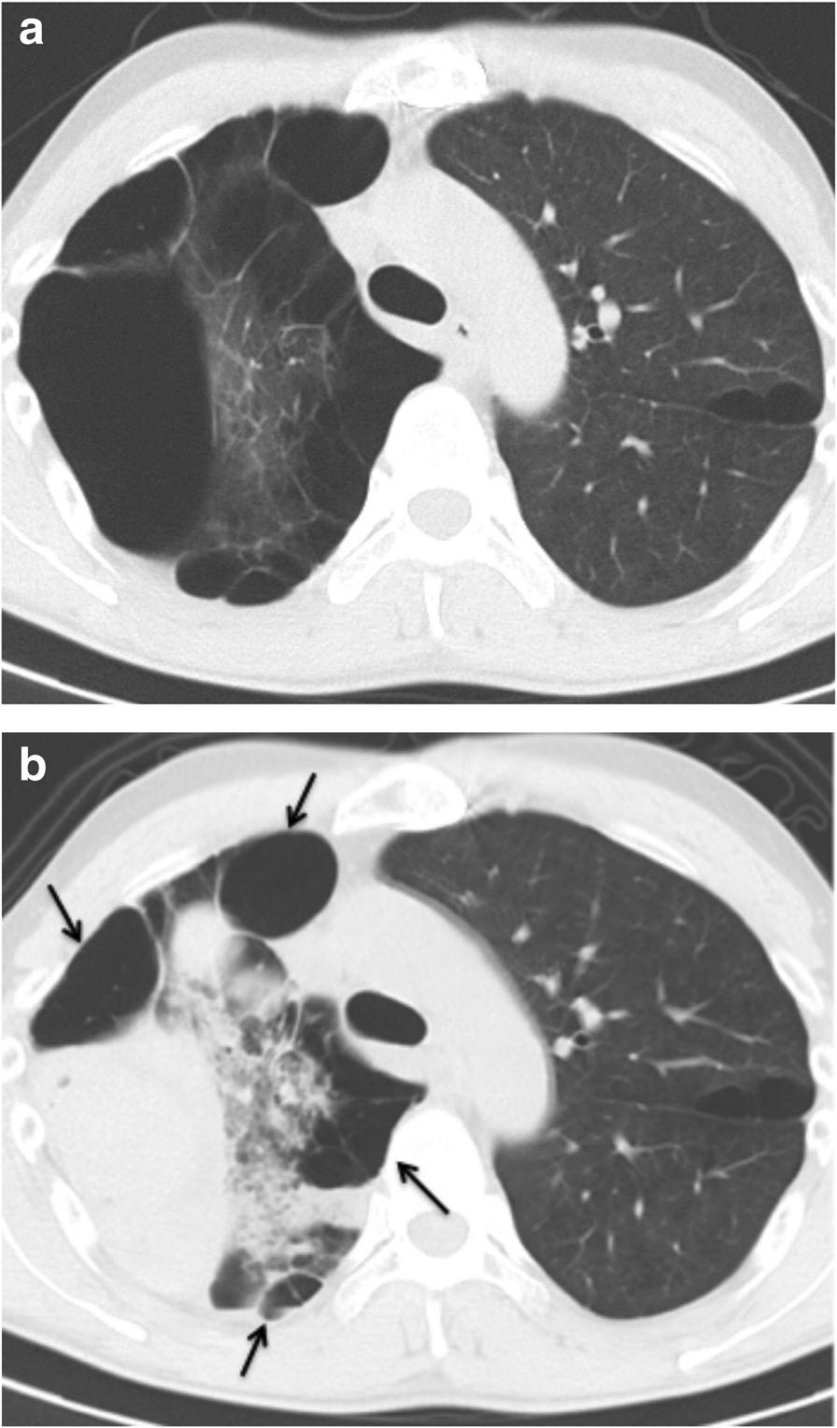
Images of chest computed tomography (CT) showing multiple cysts and thickening of the pleura around the cysts in the upper lobe before haemoptysis (a). There was a tumour shadow with a uniform density in the right upper lobe, which was demonstrated to be full of blood in the cyst (b). Another giant cyst adjacent to the tumour storing blood presented an air fluid image (b). The arrows indicate the margin of the multiple pulmonary cysts (b)
